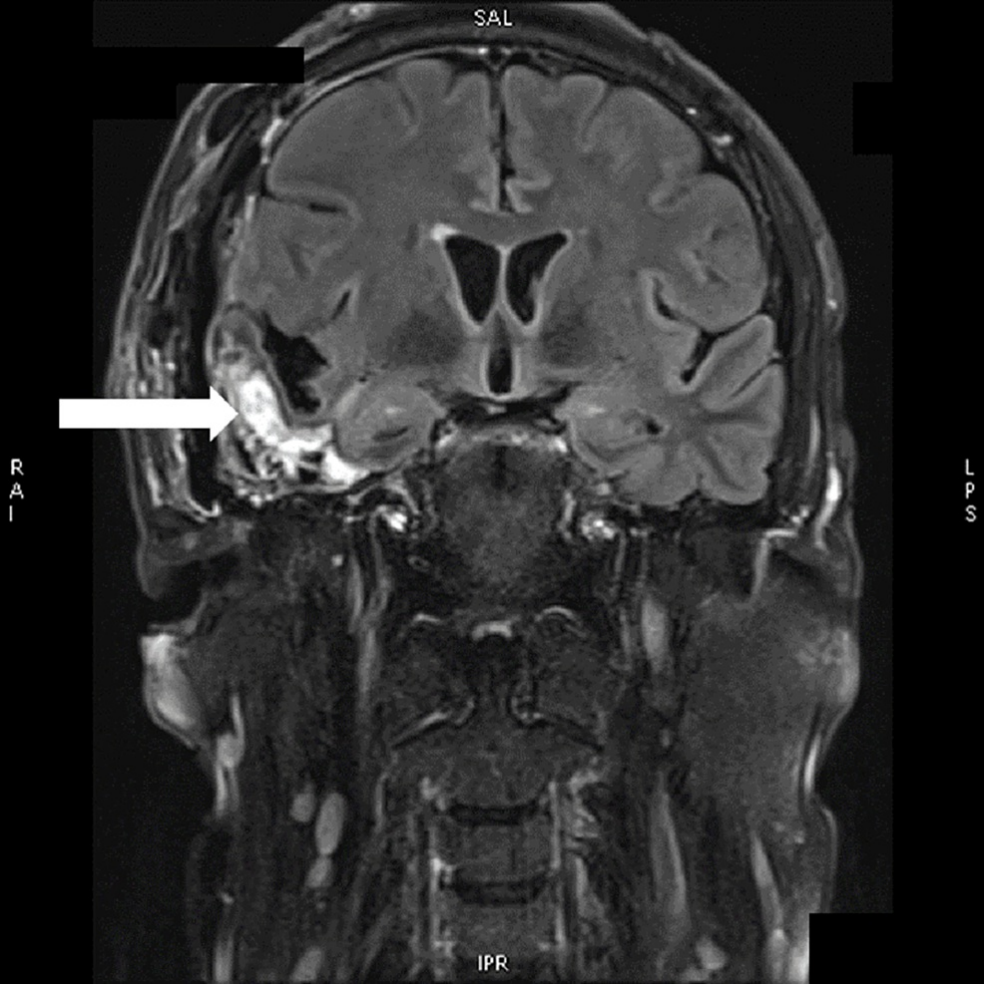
COR T2 TIRM postcontrast MRI of the brain showing postoperative changes in the right temporal lobe with residual tumor tissue (arrow) COR T2 TIRM: coronal, transverse relaxation time turbo inversion recovery magnitude; MRI: magnetic resonance imaging
